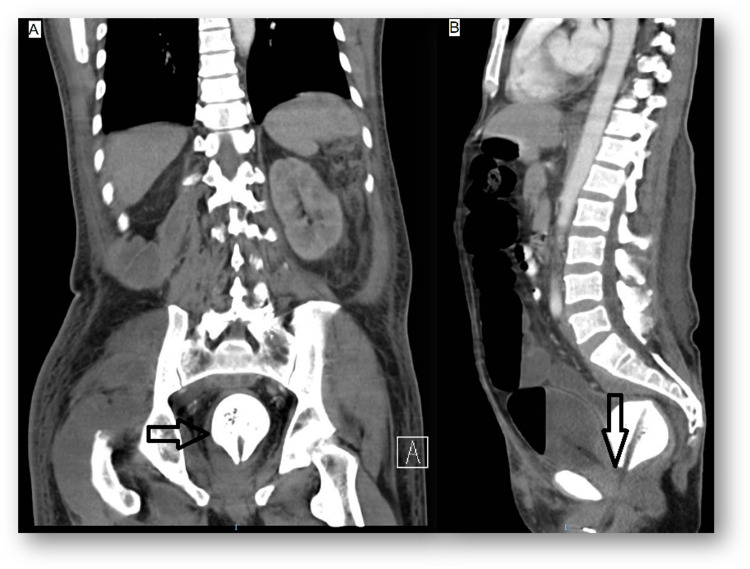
CT scan of abdomen and pelvis showing an acute inflammatory process (black arrow) with a pneumatic component, Foley catheter, and hyperdense artifact that conditions rectovesical fistula on coronary (A) and sagittal (B) planes. CT: computed tomography
